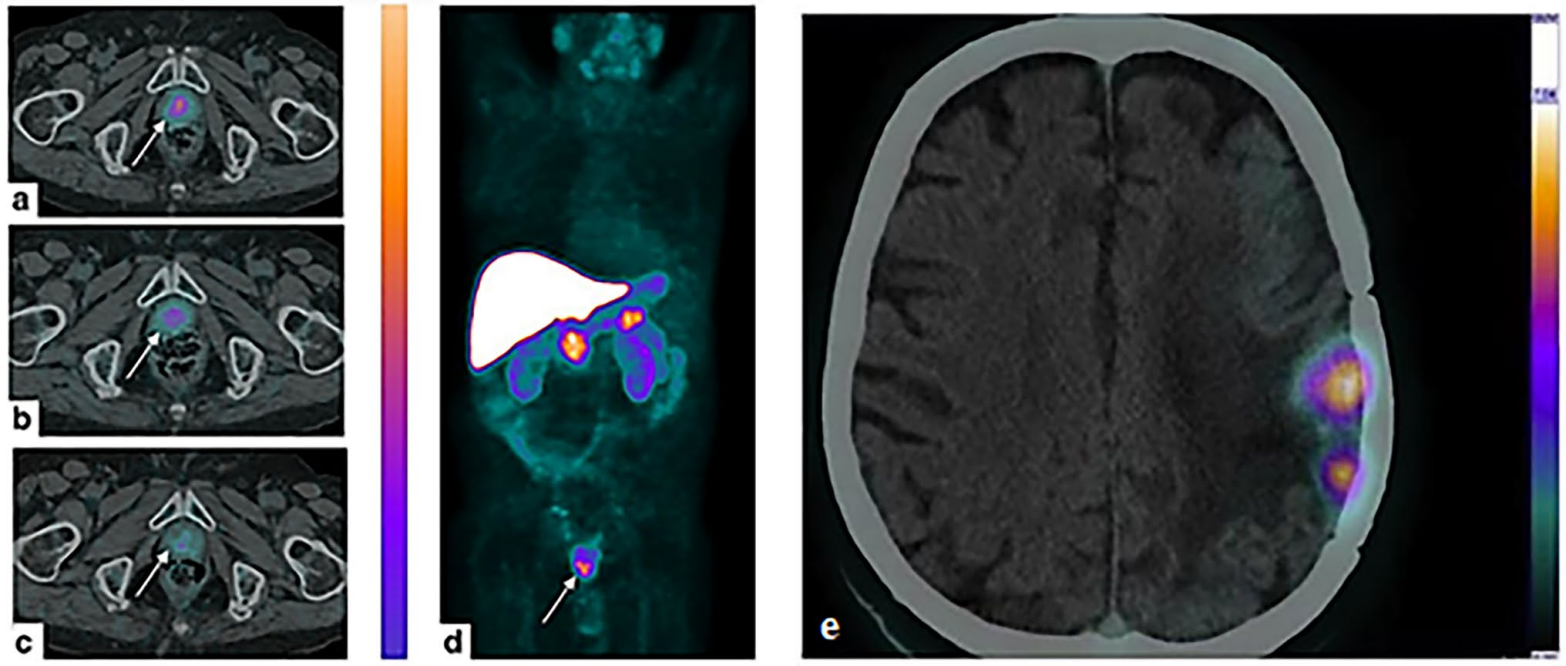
^64^CuCl_2_ PET/CT imaging: a Uptake of the tracer in the prostate apex, near the midline, 1 h after injection, b Marked reduction of tracer uptake 4 h after injection, c 24 h after injection, d Maximum intensity of tracer uptake and e Presence of cerebral glioblastoma in the brain, imaged 1 h after injection
